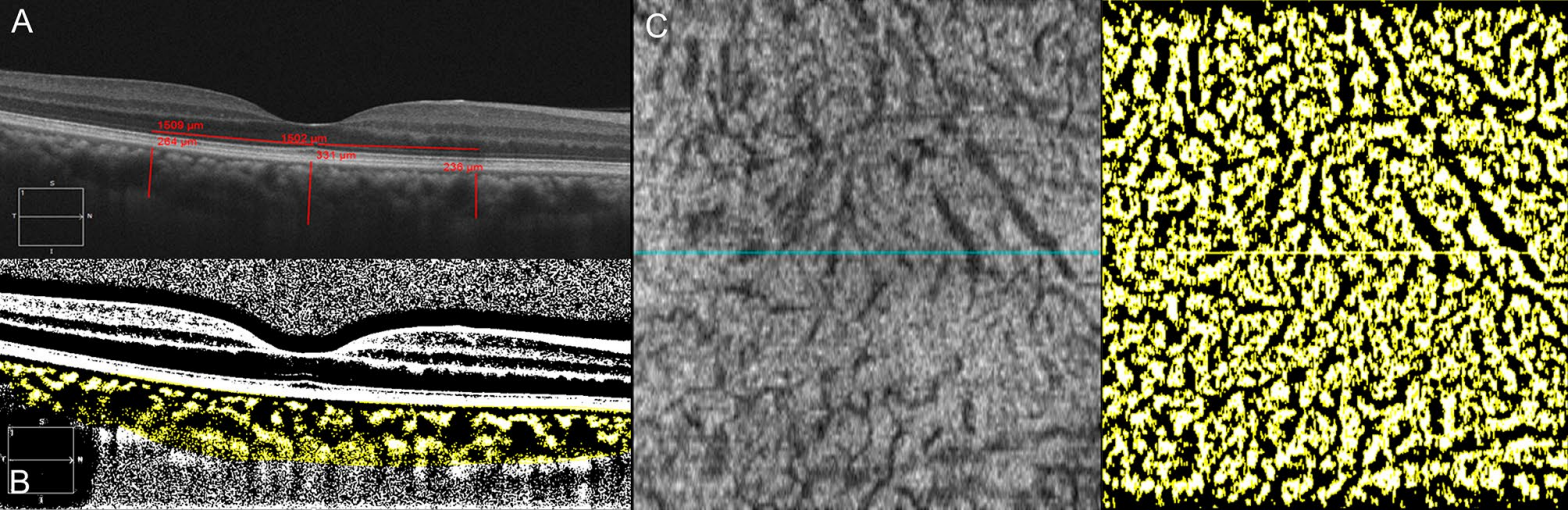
Analysis of optical coherence tomography (OCT) images. (A) Choroidal thickness (CT) was measured at three points, i.e., the center and 1500 µm nasally and temporally from the center on HD line scan OCT B-scan images that crossed the fovea. (B) Choroidal vascularity (CV) was calculated by dividing the number of pixels in the vascular area (dark pixels) within the choroid by that of the total choroidal area on the OCT B-scan image after binarization. (C) A Haller vessel slab was obtained at 50% of the total CT. CV was calculated by dividing the number of pixels in the vascular area (dark pixels) by that of the total area after binarization.
